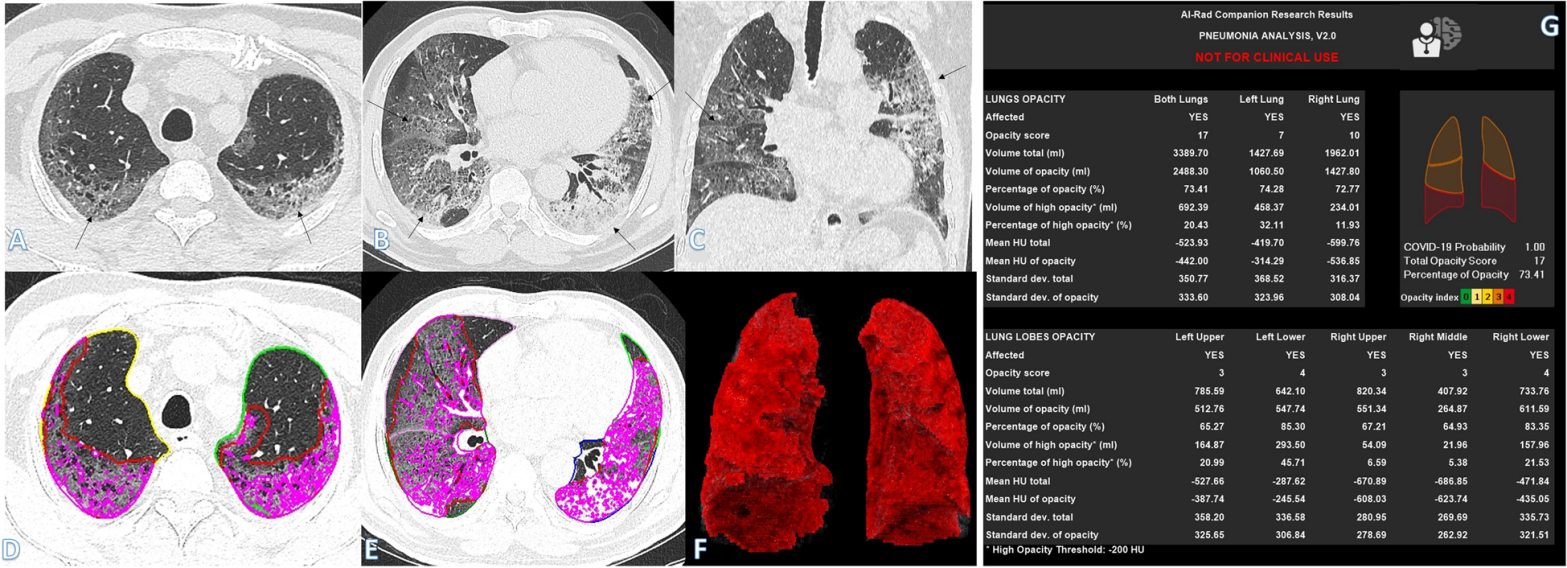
A 64-year-old male with positive COVID-19 virus. CT chest shows centrilobular emphysematous changes with bilateral diffuse ground-glass opacities with crazy-paving appearance with more involvement of both lower lobes (arrows in a–c). Total severity score = 17. Quantitative analysis by AI-Rad Companion Research CT Pneumonia Analysis was presented (d–g) with the measured parameters seen in table (g). Quantitative total opacity score was 17
